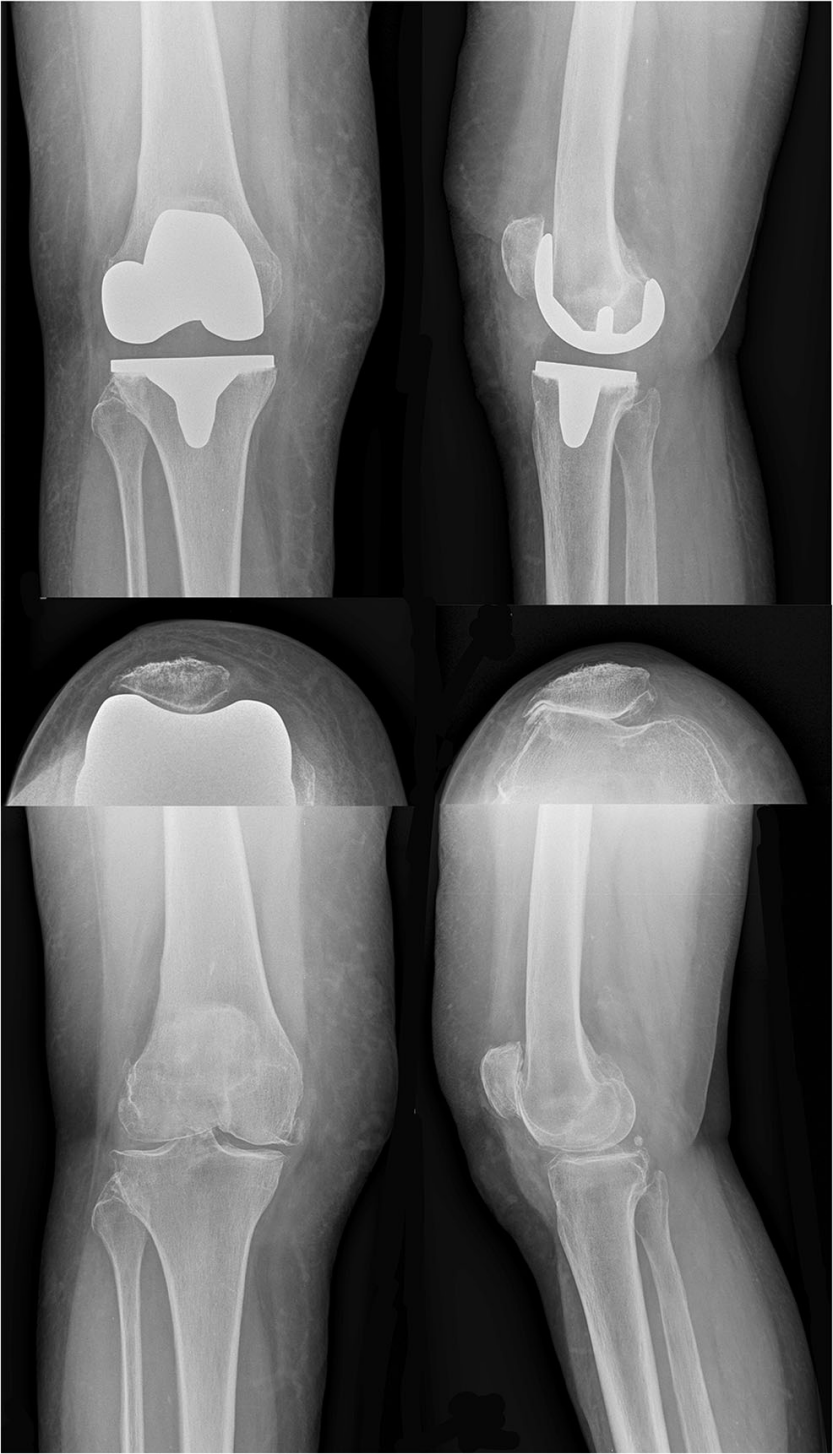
Pre- and post-operative radiographs of a patient who underwent PFC TKA. The standardised pre- and post-operative radiographs (ap, lateral, and patella tangential) of a patient who underwent PFC TKA are reported. A good TKA component position and patella height are shown
